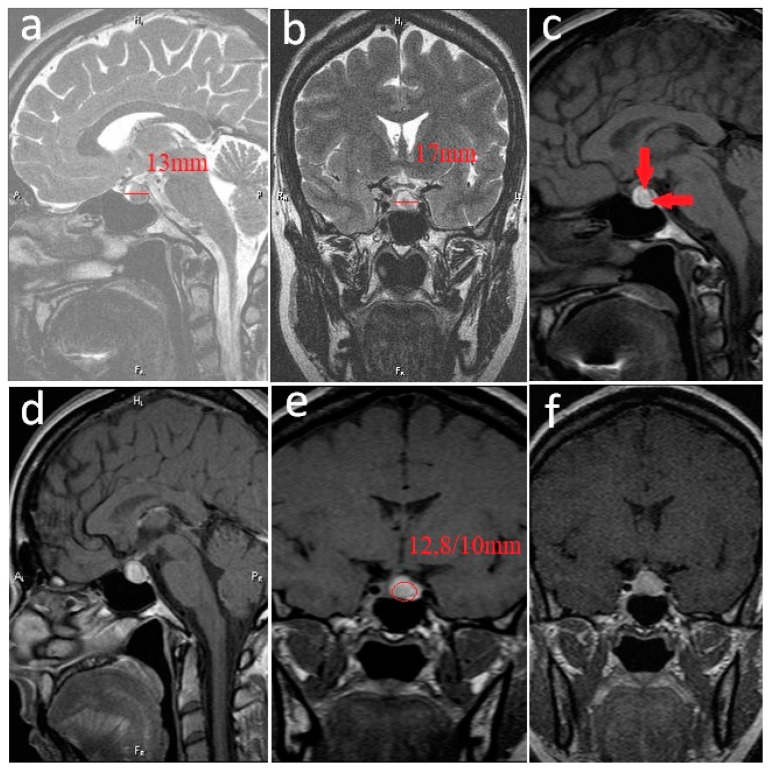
Prolactinoma with bleeding areas inside. Pituitary MRI at diagnosis. (a). Noncontrast sagittal T2-weighted image. (b). Noncontrast coronal T2-weighted image. (c). Noncontrast sagittal T1-weighted image—diffuse bleeding (hyper T1—arrows). (d). Postcontrast sagittal T1-weighted image. (e). Postcontrast coronal T1-weighted image. (f). Postcontrast coronal T1-weighted image. Pituitary fossa—anteroposterior diameter: 13 mm; transverse diameter: 17 mm; oval-shaped lesion 12.8/10 mm; heterogeneous high-signal T2-weighted (a,b); T1-weighted (c,d), located in the adenohypophysis with high signal in T1; weighted areas corresponding to the subacute intratumoral hemorrhage due to the presence of methemoglobin, heterogeneous enhancement (e,f), extending superiorly to the pituitary fossa (b,d,e,f) with mild compression of the optic chiasma (b,d,f).
